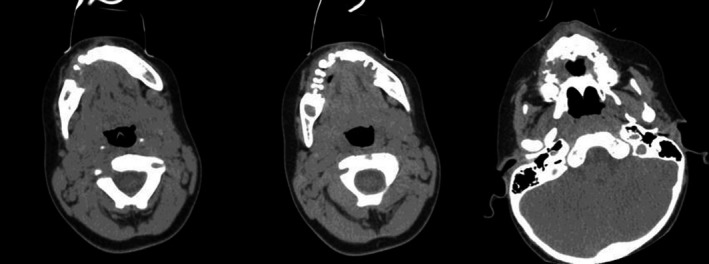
Head and neck CT scan shows irregular destructive radiolucent lesions in the mandible and the maxillary bones with soft tissue component
